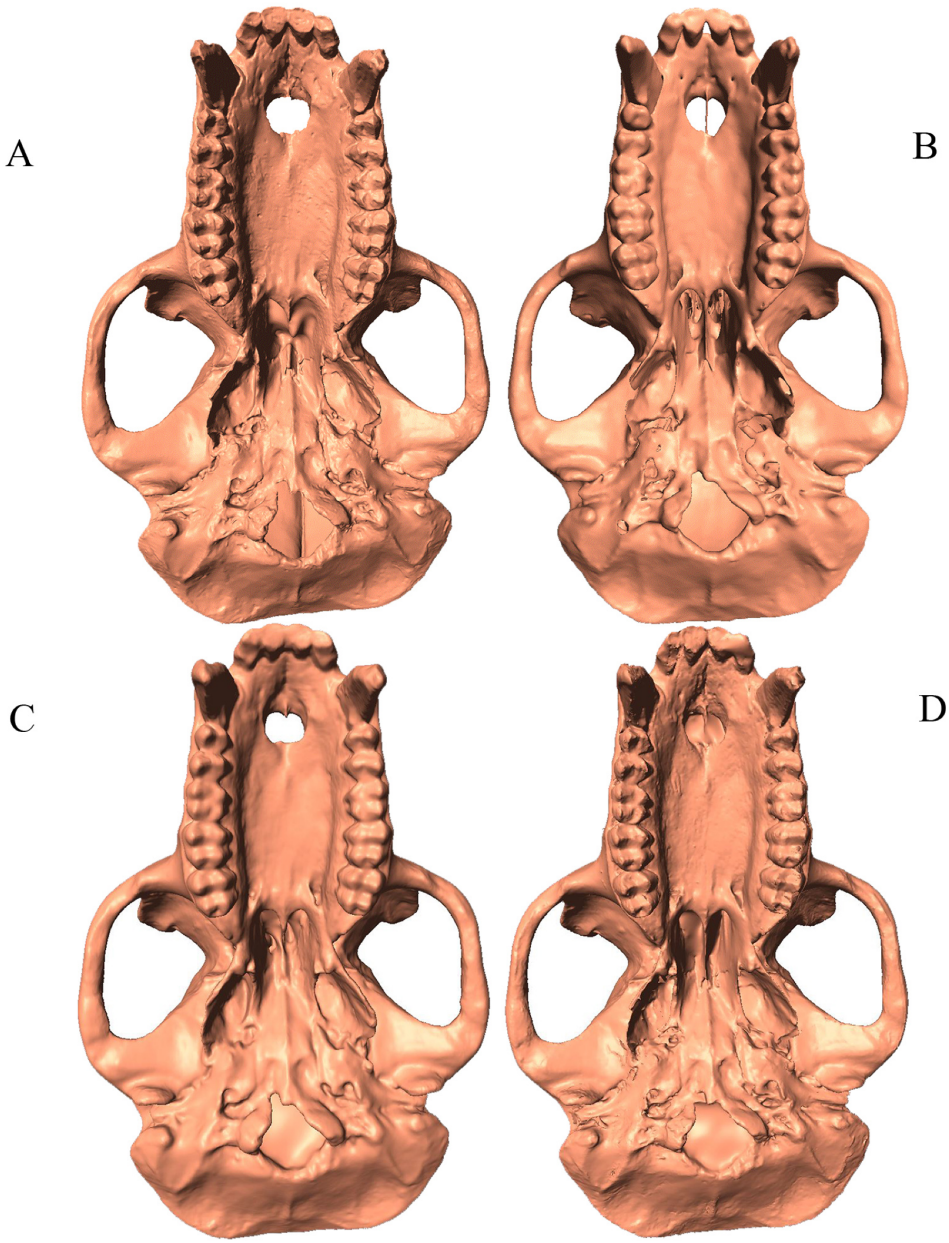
Scan comparison inferior view of *Macaca thibetana* (AMNH 129). (A). Breuckmann OptoTOP-HE; (B) GE phoenix v|tome|x s240 CT scan; (C) Minolta Vivid 910; (D) NextEngine 3D Scanner HD.
